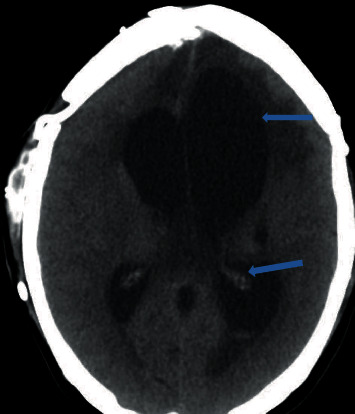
CT head showing moderate enlargement of the ventricular system with left-sided intraventricular hemorrhage (blue arrows).
